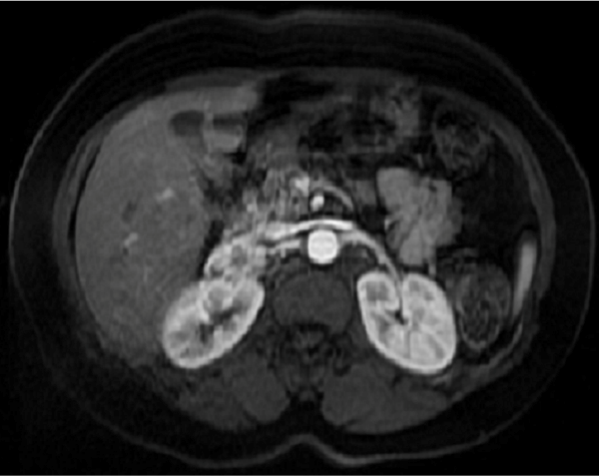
Magnetic resonance imaging identified a left suprarenal mass
